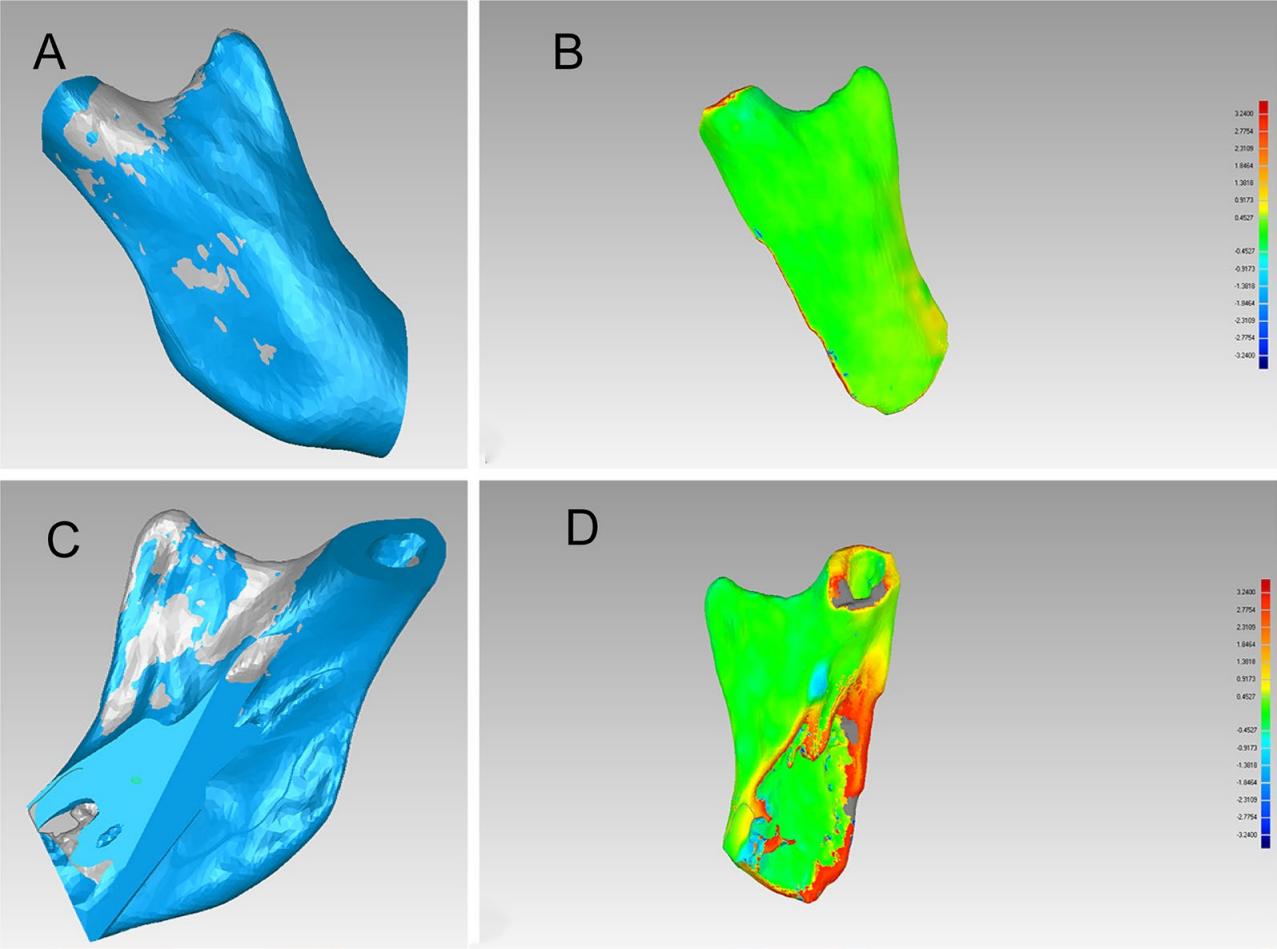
Evaluation of the accuracy of condylar osteotomy guide. a, c Superimposition of the virtual plan (blue) and postoperative CT scan at 3 days after surgery (grey); b, d the postoperative accuracy study of condylar osteotomy guide using the Geomagic Studio 2013 software
